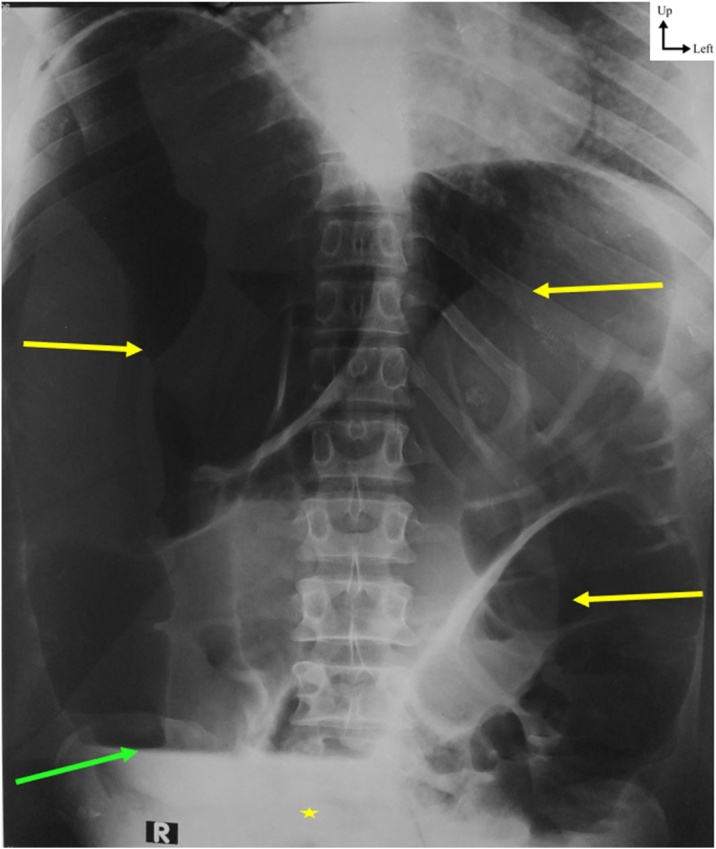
Plain abdominal radiography showing diffuse gas distension of the colon (yellow arrows) with hydro-aeric levels (green arrow) and without gas in the pelvis (yellow star).
